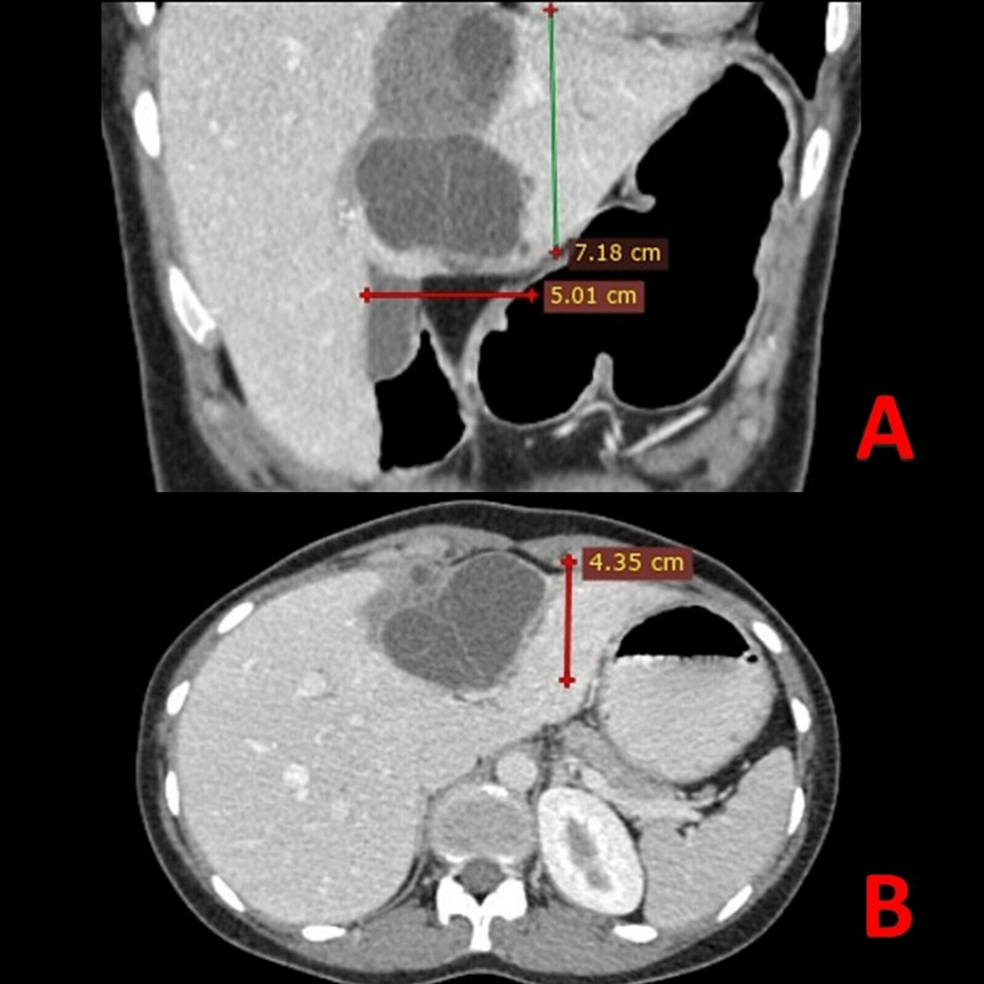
Venous phase of contrast-enhanced CT scan (CECT) (A) Coronal plane, and (B) Axial plane, showing a multi-cystic 7 x 5 x 4.3cm lesion in the segment IVa and IVb of the left lobe of the liver suggestive of hydatid cyst.
